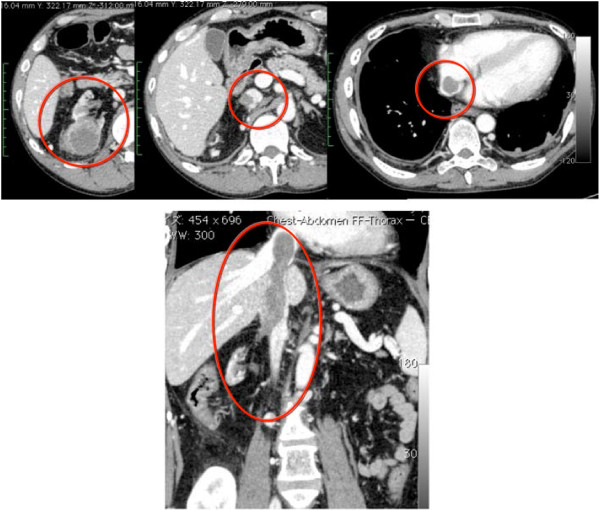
Computed tomography scan after 10 months of preoperative sunitinib therapy. The level of tumor thrombus was evaluated as IV according to Novic’s classification; there were no changes from before sunitinib therapy. However, the size of the primary tumor was reduced by 33%, and that of the tumor thrombus decreased by 39.5%. The red circle indicates the primary tumor and thrombus.
